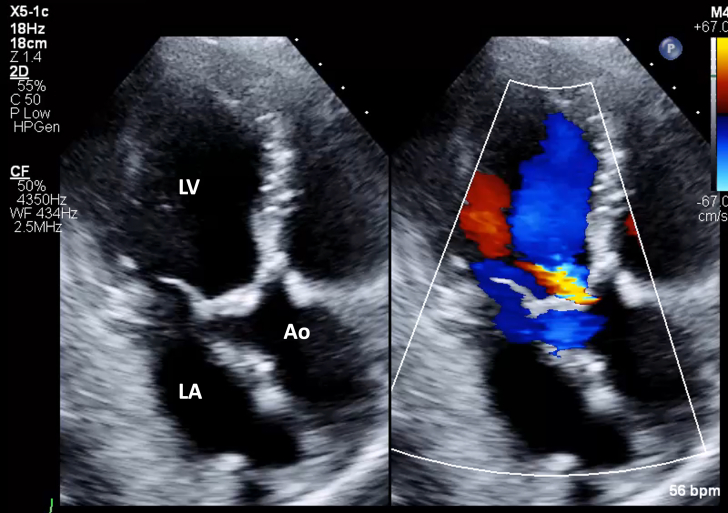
Apical 3-Chamber View Evaluation for Aortic Stenosis With and Without Color Doppler Apical 3 chamber: color Doppler on aortic valve to evaluate for aortic regurgitation and a stenotic mosaic color flow pattern caused by an outflow obstruction. Abbreviations as in Figure 2.
